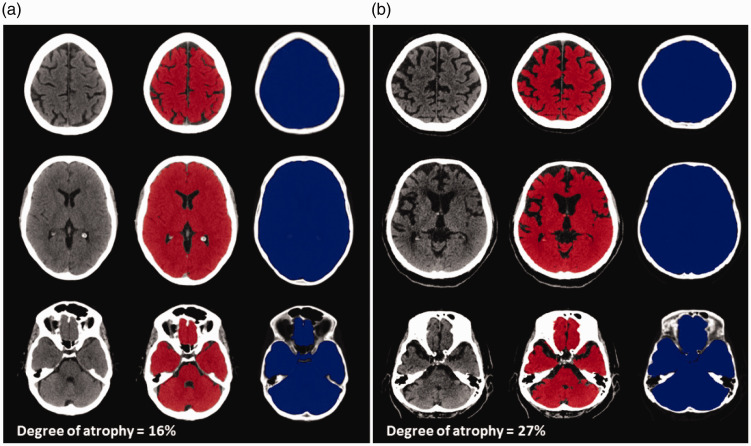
Assessment of brain atrophy. Examples of axial non-contrast CT images of two patients with total brain volume (TBV) masks shown in red and intracranial volume (ICV) masks shown in blue. (a) A 43-year-old female patient presenting with a left M2 middle cerebral artery occlusion. (b) An 81-year-old male patient presenting with a left intracranial carotid artery occlusion.
